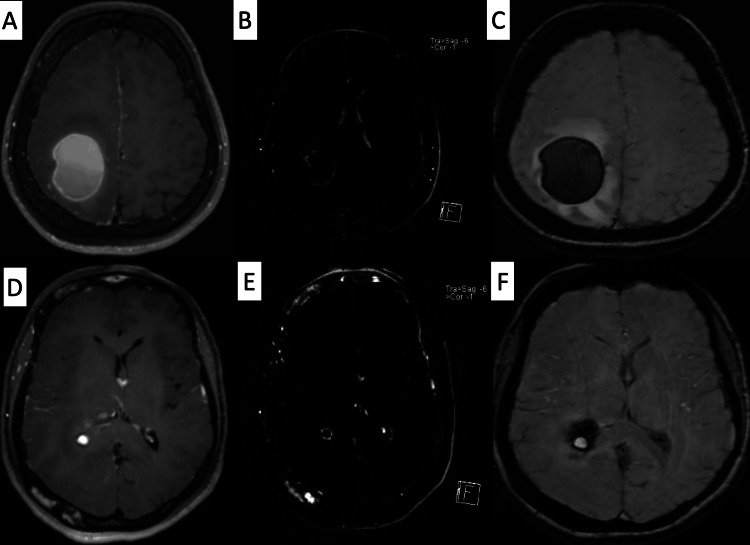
MRI T1 images post contrast A and D are T1-weighted images post-contrast with subtraction images (B and E) at two different levels of the same lesion showing a thin rim of enhancement. On susceptibility-weighted images (C and F), the lesion contained a susceptibly effect and a relatively higher rim of susceptibility.
